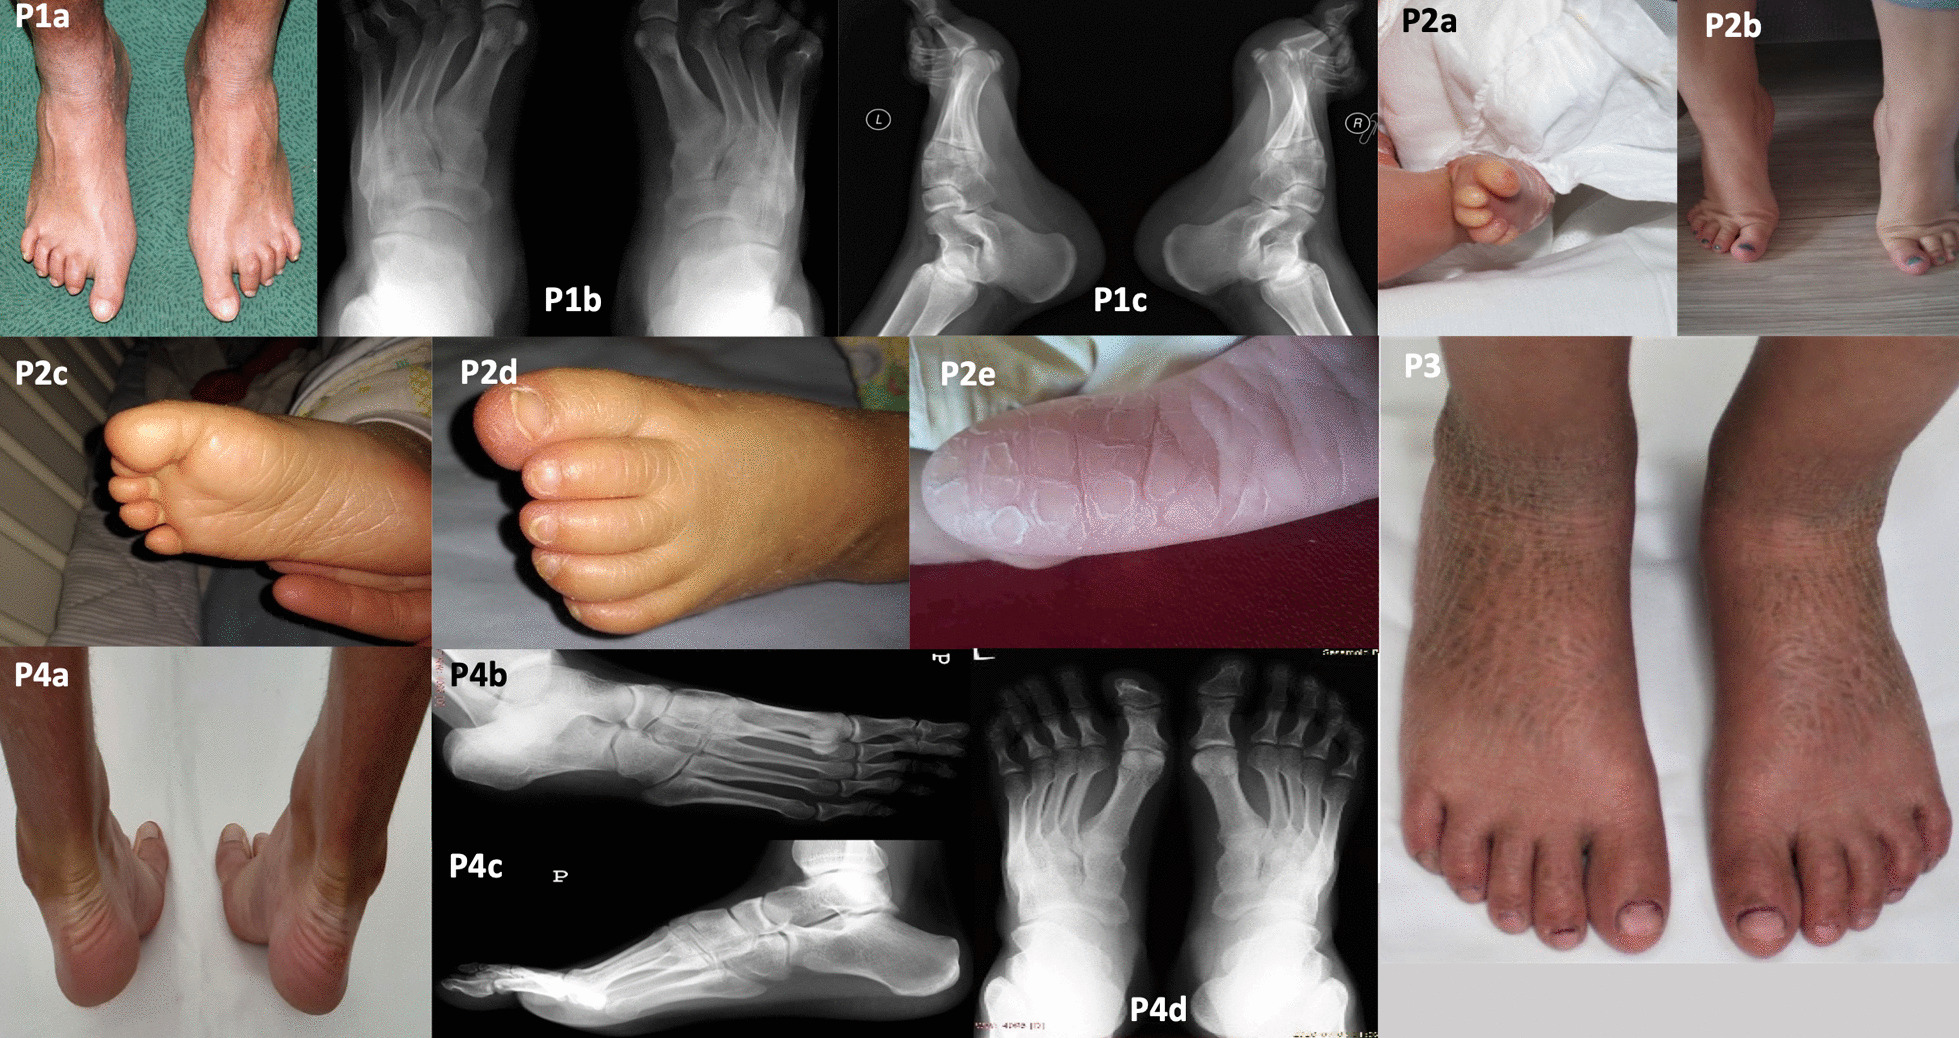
Patients 1–4 with autosomal recessive congenital ichthyosis. Pes cavus, pes varus, toe contractures, hollow feet, widened forefeet, claw toes II–V, and varus heels rotated inwards are visible on a photograph (P1a) and radiographs (P1b, P1c). Toe contractures at birth (P2a). Morphologically normal feet, but early signs of claw and varus toes (toes III–V overlapping) were seen when standing on tiptoes (P2b–c). Skin cracks on the soles (P2d–e) with plaques and fissures (P2f). Normal feet (P3). Normal feet clinically (P4a) and radiologically (P4b–c)
